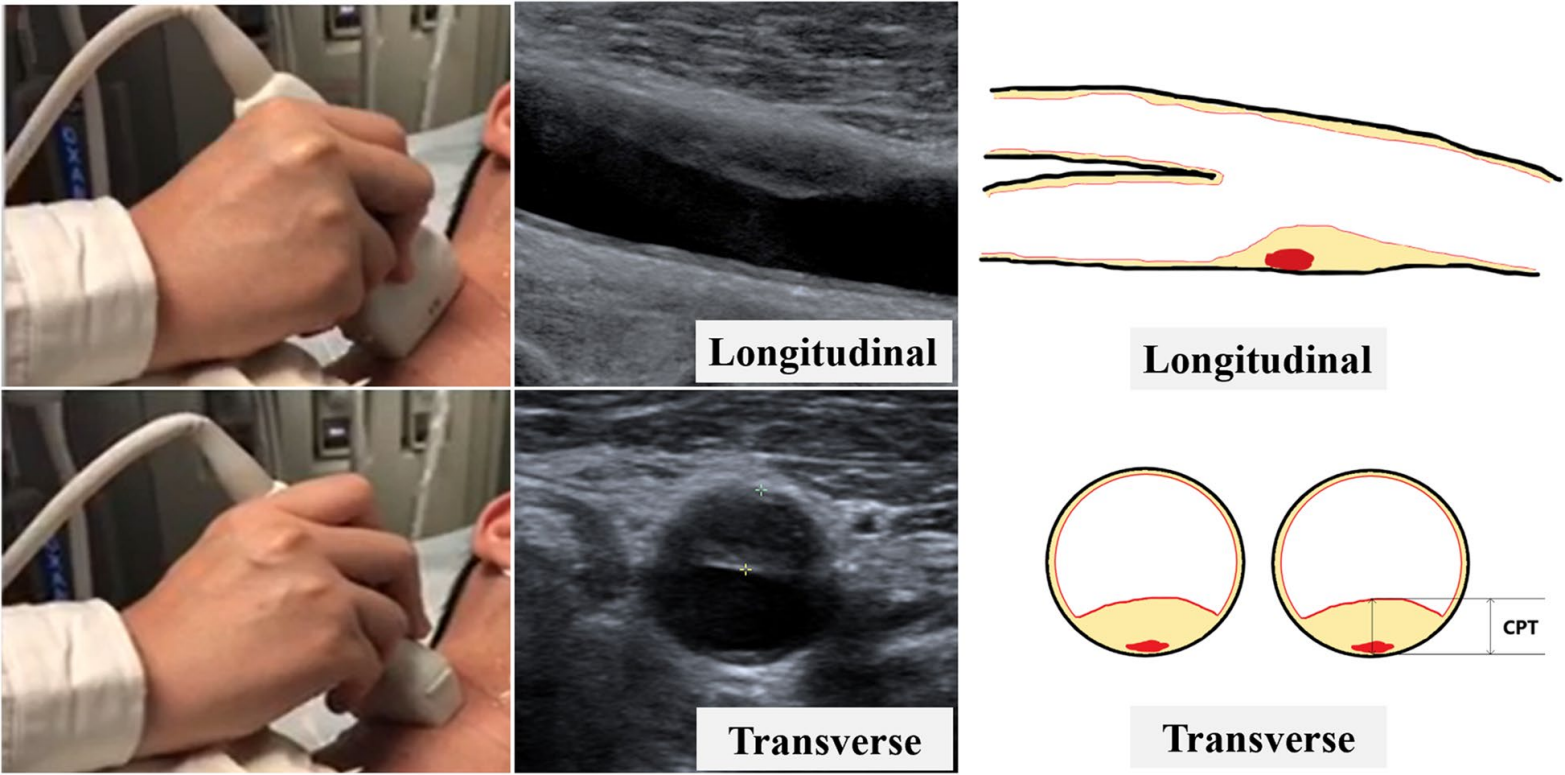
Carotid ultrasound imaging and carotid plaque thickness (CPT) measurement
